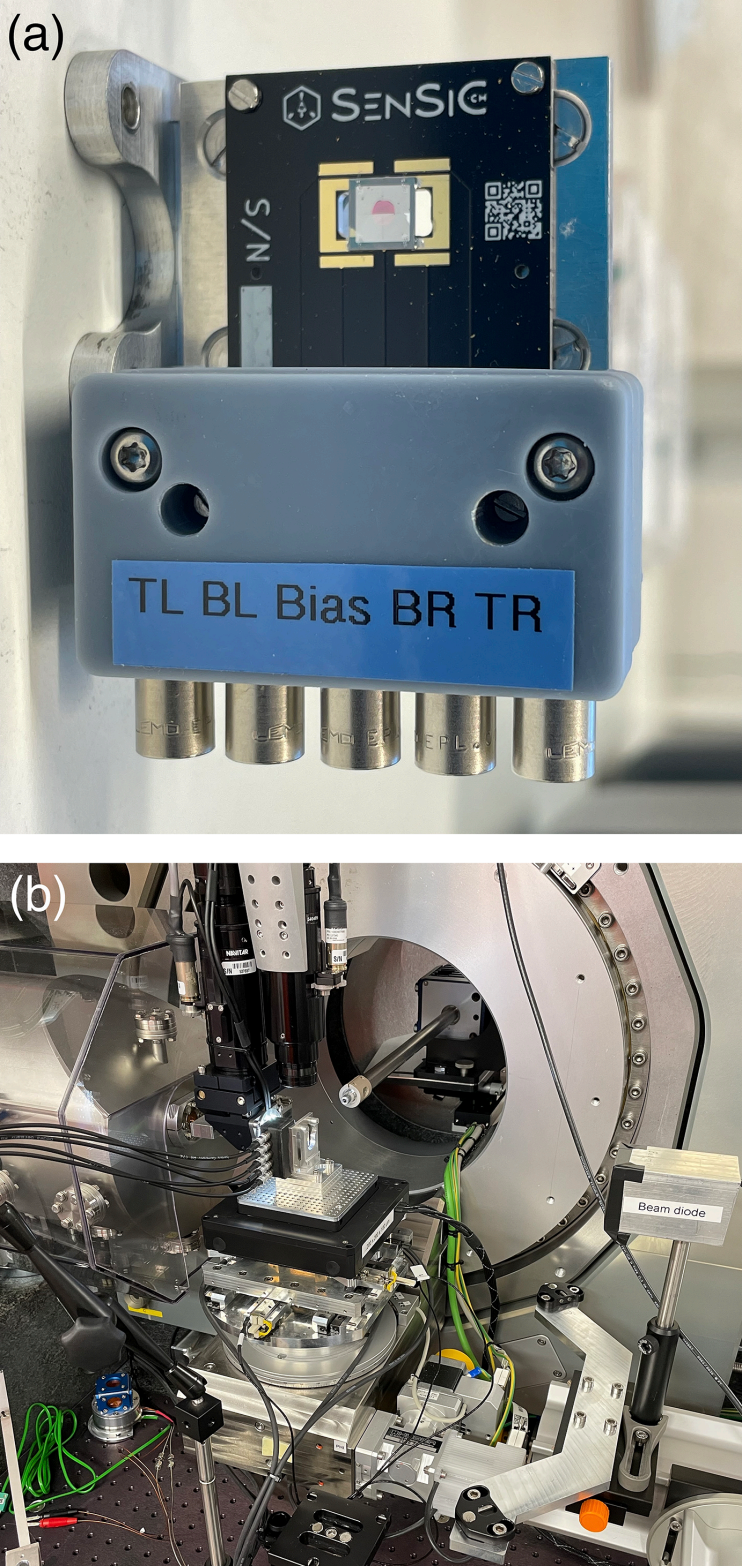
Photographs of the experimental setup at NanoMAX beamline. The SiC sensor (*a*) is mounted in the hard X-ray monochromatic nanoprobe experimental station (*b*).
